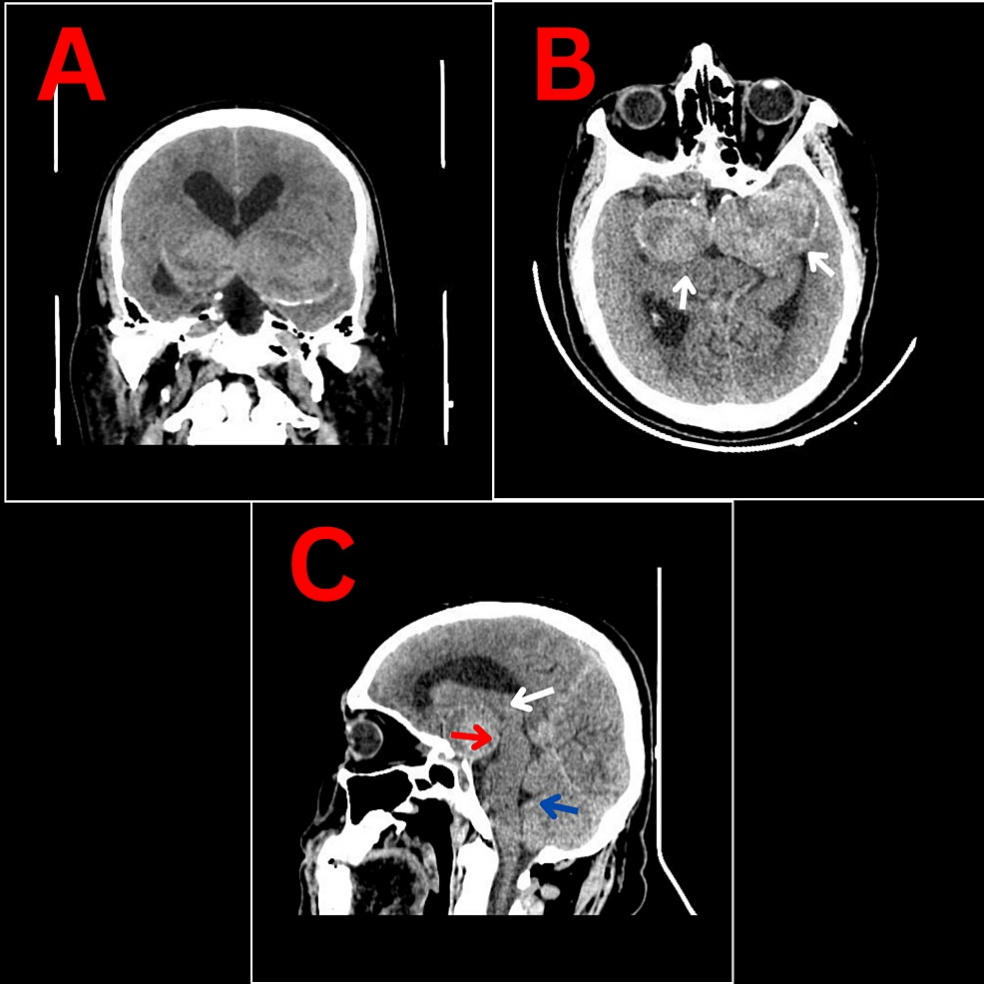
Head CT Scan without contrast showing the bilateral huge supraclinoid carotid aneurysms. A: Coronal cut. B: Axial cut showing the giant bilateral partially thrombosed supraclinoid carotid aneurism holding calcification (arrow) surrounded by perilesional edema causing compression on midbrain and hydrocephalus by obstructing the third ventricle. C: Sagittal view showing the aneurysms compressing the thalamic and hypothalamic structures (white arrow), as well as the superior anterior aspect of the brainstem (red arrow), the 4th ventricle not dilated (blue arrow).
